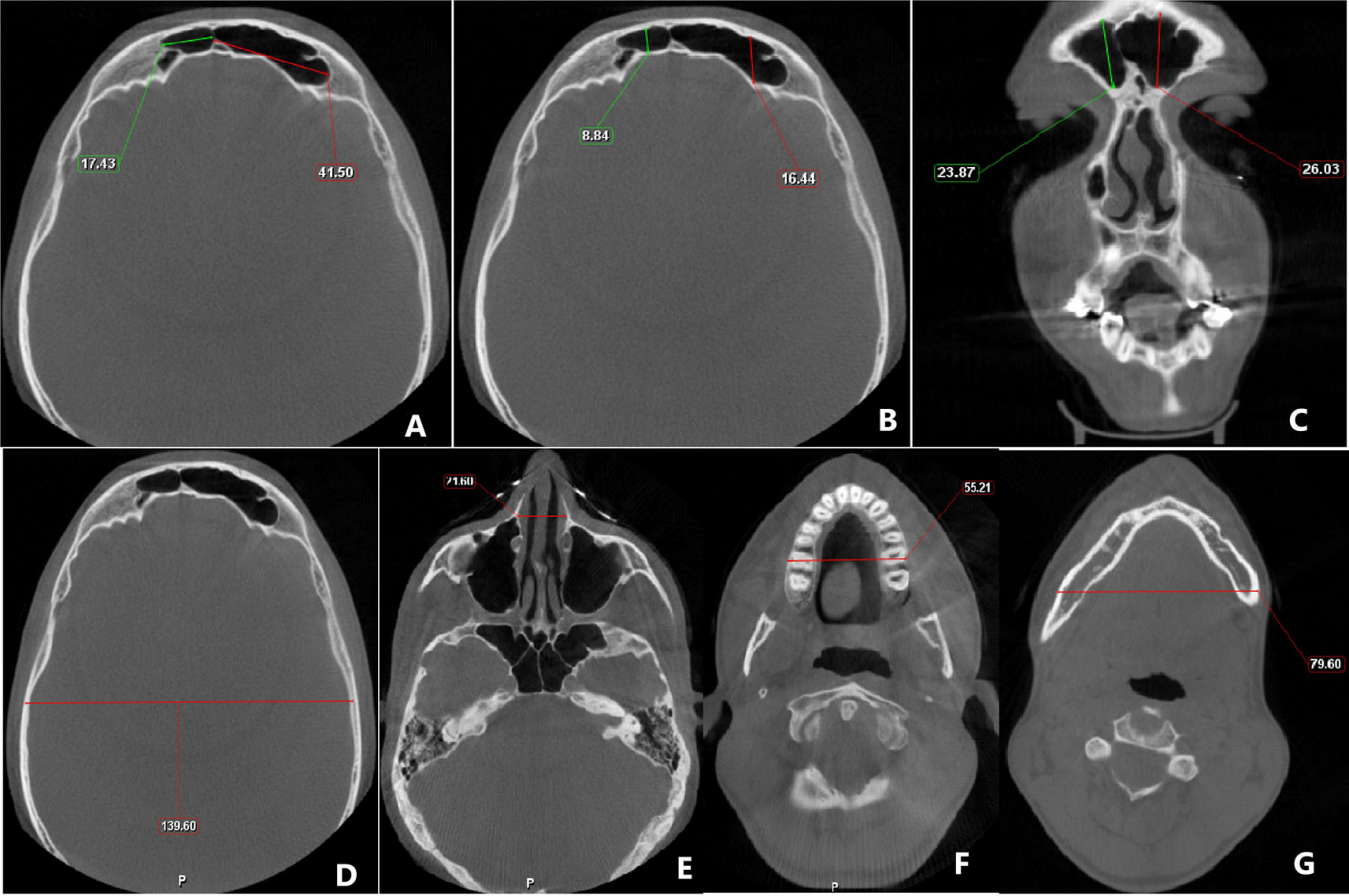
Cone-beam computed tomography image showing the measurements of A) mesio-distal dimension of frontal sinus; B) antero-posterior measurement of frontal sinus; C) supero-inferior measurement of frontal sinus; D) cranial width; E) nasal width; F) maxillary width; G) mandibular width.
